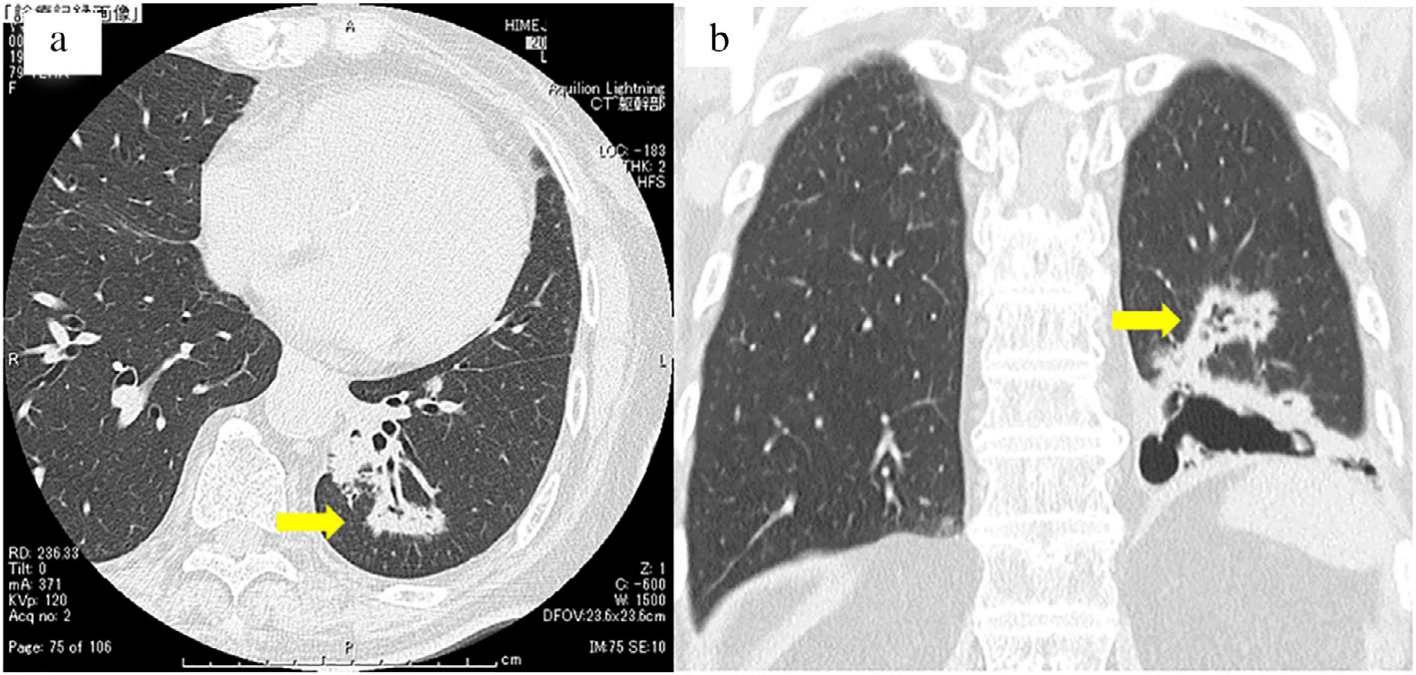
Chest computed tomography (CT) findings. (a) A nodule is seen in the left lower lobe (axial view). (b) A nodule measuring 35 mm in diameter and pulmonary bullae adjacent to the tumor can be seen (coronary view)
